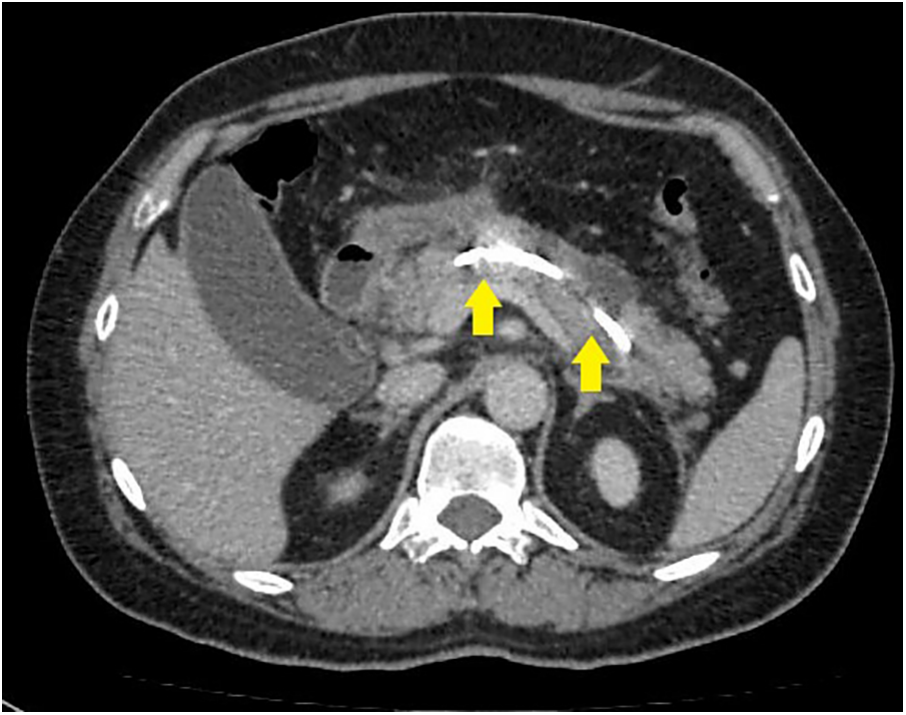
CT image after endoscopic transpapillary stent placement in the main pancreatic duct in patients with necrotizing pancreatitis (stents in the main pancreatic duct and common hepatic duct are indicated by arrows).
